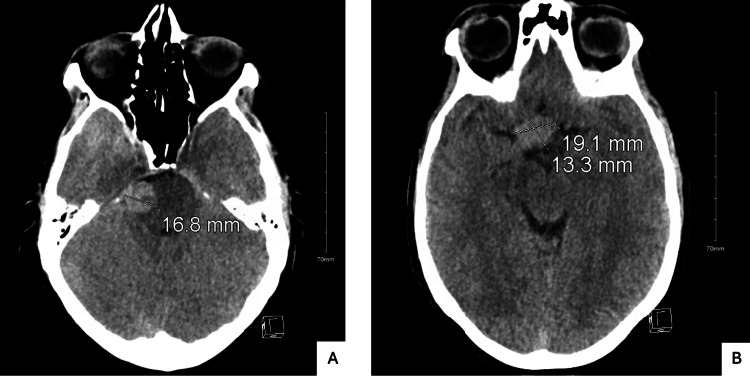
Non-Contrast CT of the Brain Revealing a New Pontine and Suprasellar Mass Non-contrast CT of the brain obtained one year later. Panel A shows a new mass adjacent to the right pons measuring 16.8 mm. Panel B reveals a suprasellar mass measuring 19.1×13.3 mm, which was not present on prior imaging. CT: computed tomography.
